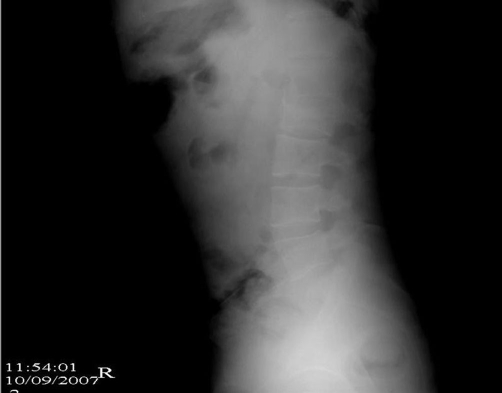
Lateral X-Ray of the case.
